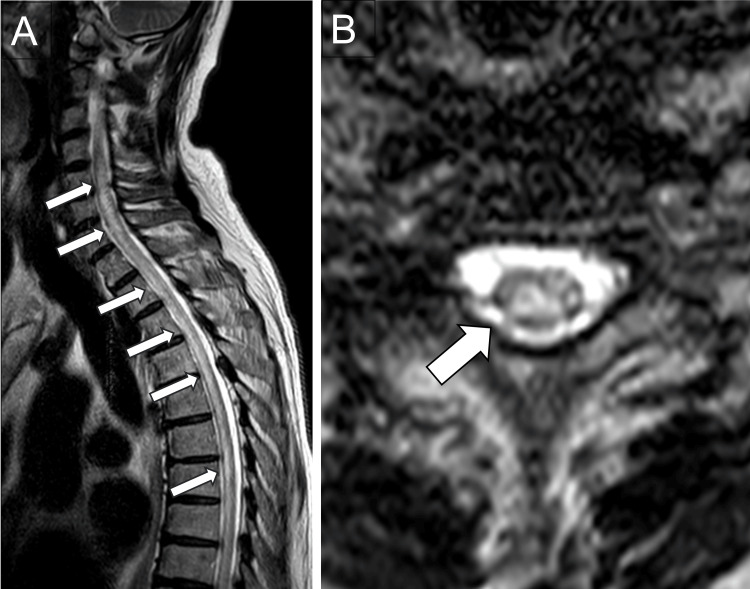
Spinal MRI on admission Sagittal (A) and axial (B) T2-weighted images show an extensive longitudinal transverse myelitis lesion (arrows) in the spinal column from the upper cervical to the thoracic region. MRI, magnetic resonance imaging
